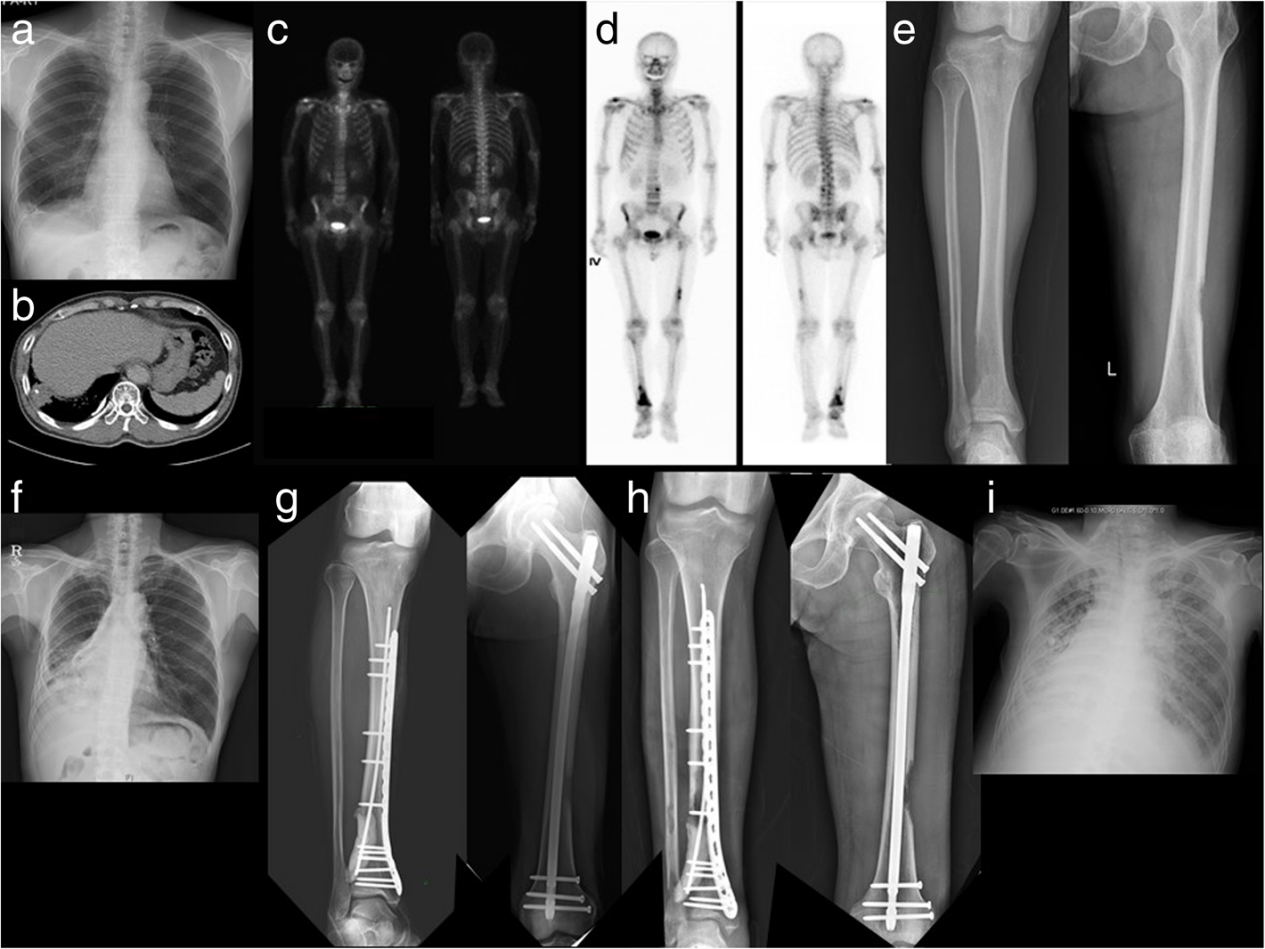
A 66-year-old man who visited our hospital for hemoptysis. a Chest X-ray showed right costo-phrenic angle blurring. b Chest CT scan showed a 4 cm-sized mass. Squamous cell carcinoma was diagnosed by bronchoscopic biopsy. c At the time of diagnosis, the stage was T3N2M0. d At follow-up, WBBS was performed at 20 months of diagnosis, and hot-uptake was observed at the 2nd Lumbar body, left distal femur diaphysis and right distal tibia diaphysis. e The osteolytic lesions are observed in the lateral cortex of the left femur diaphysis and lateral cortex of right tibia diaphysis, suggesting skeletal metastases. f In chest X-ray, the haziness was increased at the right mid and lower lung field. g Curettage, flexible intramedullary nailing, plate fixation and bone cementing were performed on the right distal tibia diaphysis metastasis. Interlocking intramedullary nailing was performed on the left femur distal diaphysis metastasis. Additional postoperative radiation therapy was performed, and chemotherapy was continued. h Ten months after surgery, there was a slight increase in the size of the osteolytic lesions around the surgical sites, but full weight bearing without pain was possible. i The patient expired from pneumonia associated with lung cancer
